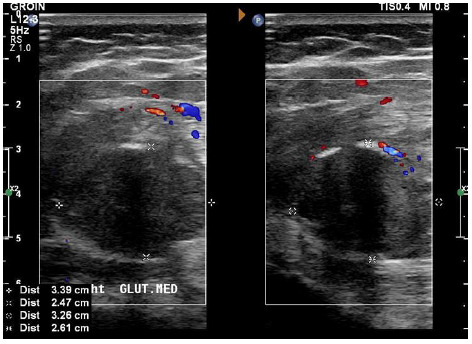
Doppler Ultrasound - Mass (70 x 45 x 35mm) identified within the right gluteus musculature. Mildly increased vascularity seen within the mass and at the periphery. Possible calcifications seen within the lesion.
